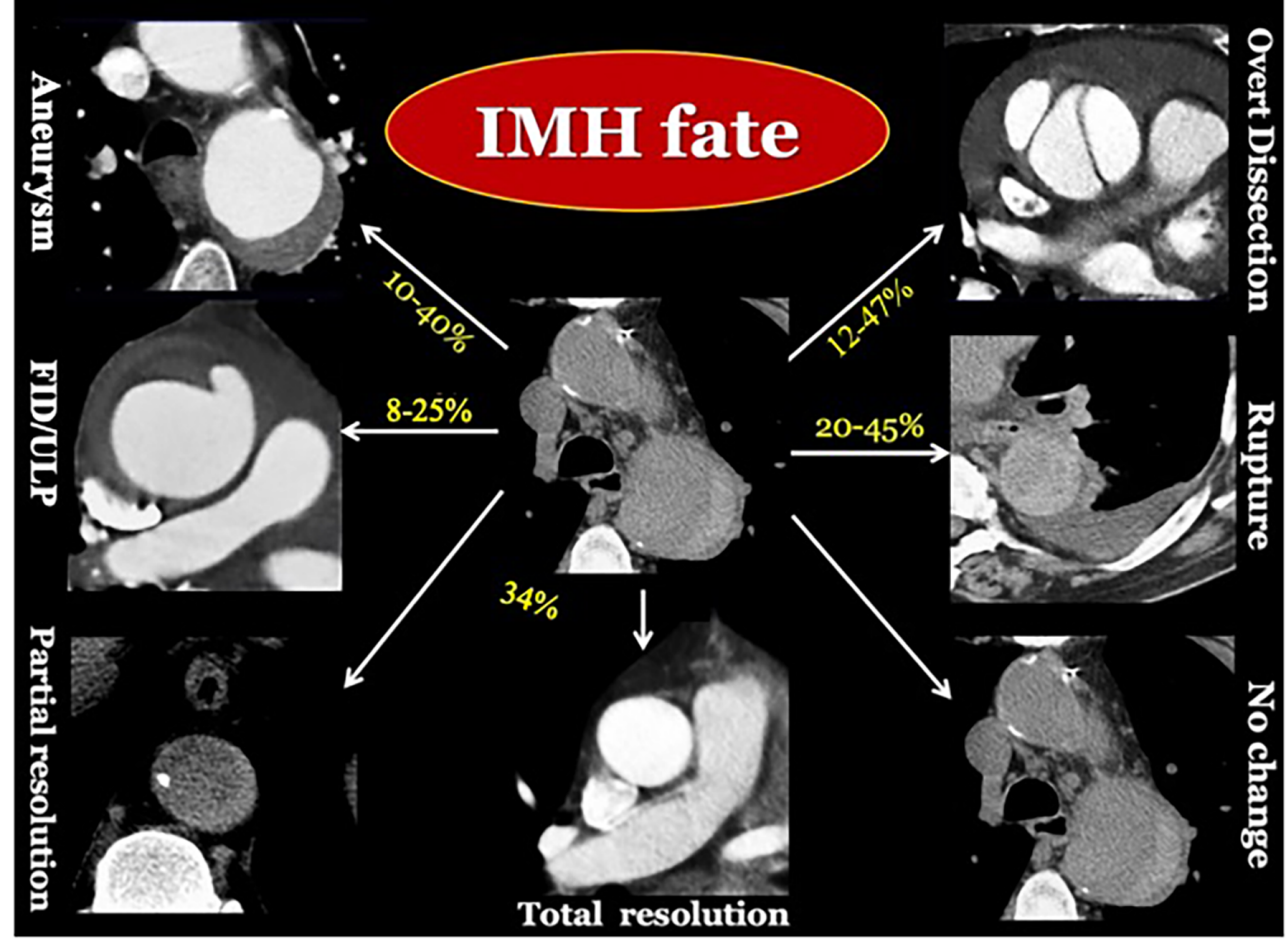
Longitudinal CT imaging of IMH evolving patterns. IMH is a vulnerable dynamic condition, with unpredictable late fate (16, 18, 57, 61).
